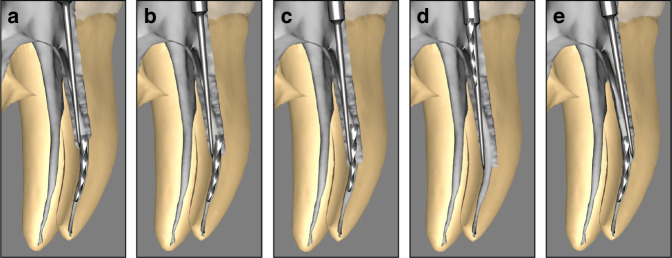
Ultrasonic technique for retrieving SI. a Ultrasonic tip removes dentin on one side of the SI. b Ultrasonic tip wedges between the SI and the root canal wall. c Loosening of the SI. d SI “jumps out” of the canal orifice. e The ultrasonic tip removes thicker dentin on one side of the SI
